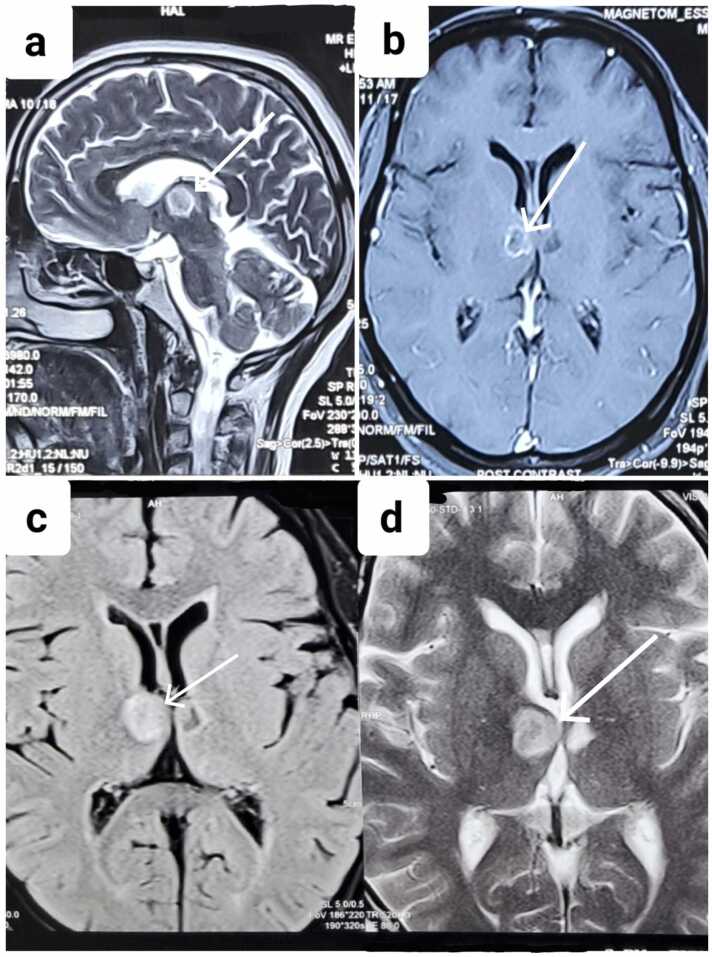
MRI features of thalamic tuberculoma of Case 5 (a) Sagittal T2-weighted image showing a hyperintense thalamic lesion (arrow). (b) Axial post-contrast T1-weighted image demonstrating ring enhancement of the thalamic lesion (arrow). (c) Axial FLAIR image showing hyperintense thalamic lesion with central hypointensity (arrow). (d) Axial T2-weighted image showing hyperintense thalamic lesion with perilesional edema (arrow).
